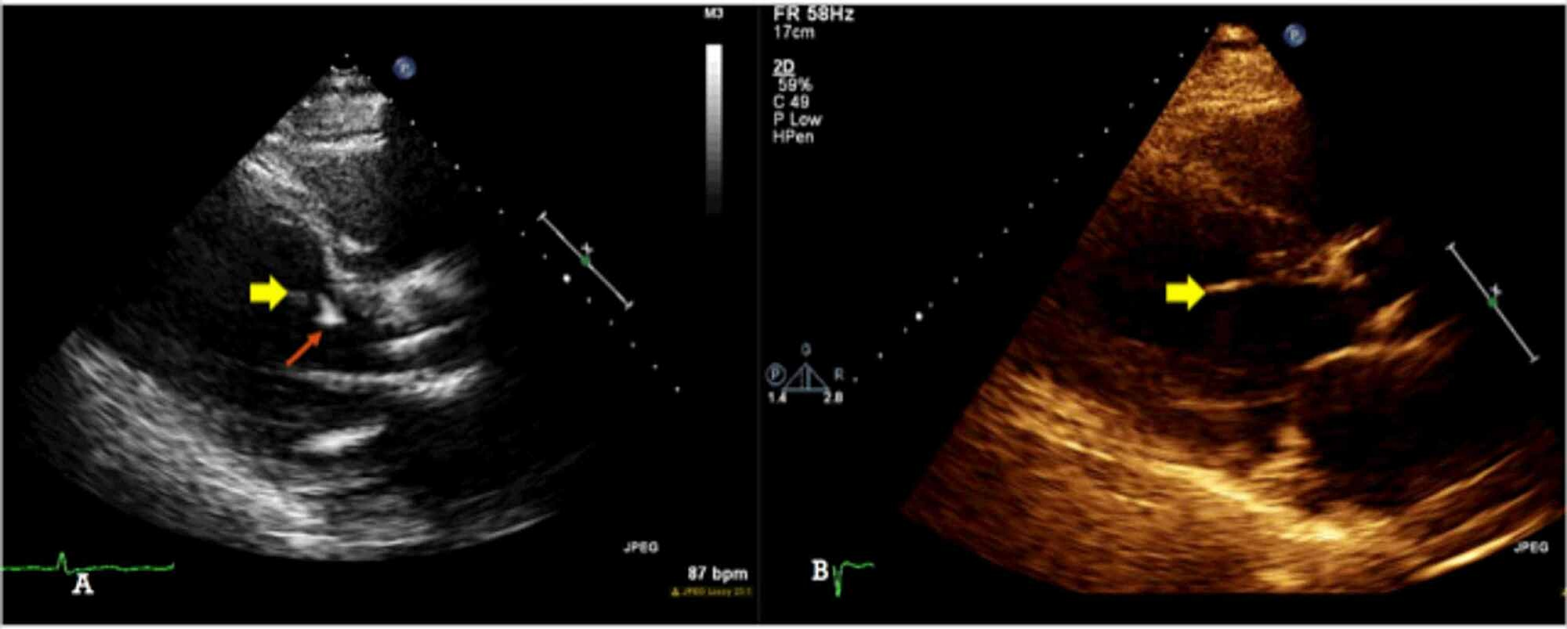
Comparison between current and prior transthoracic echocardiogram A: current transthoracic echocardiogram shows a linear structure (yellow arrow) next to the echodensity (red arrow). B: prior echocardiogram from one year ago shows a hyperechoic false tendon in the same location as the linear structure from the current echocardiogram.
